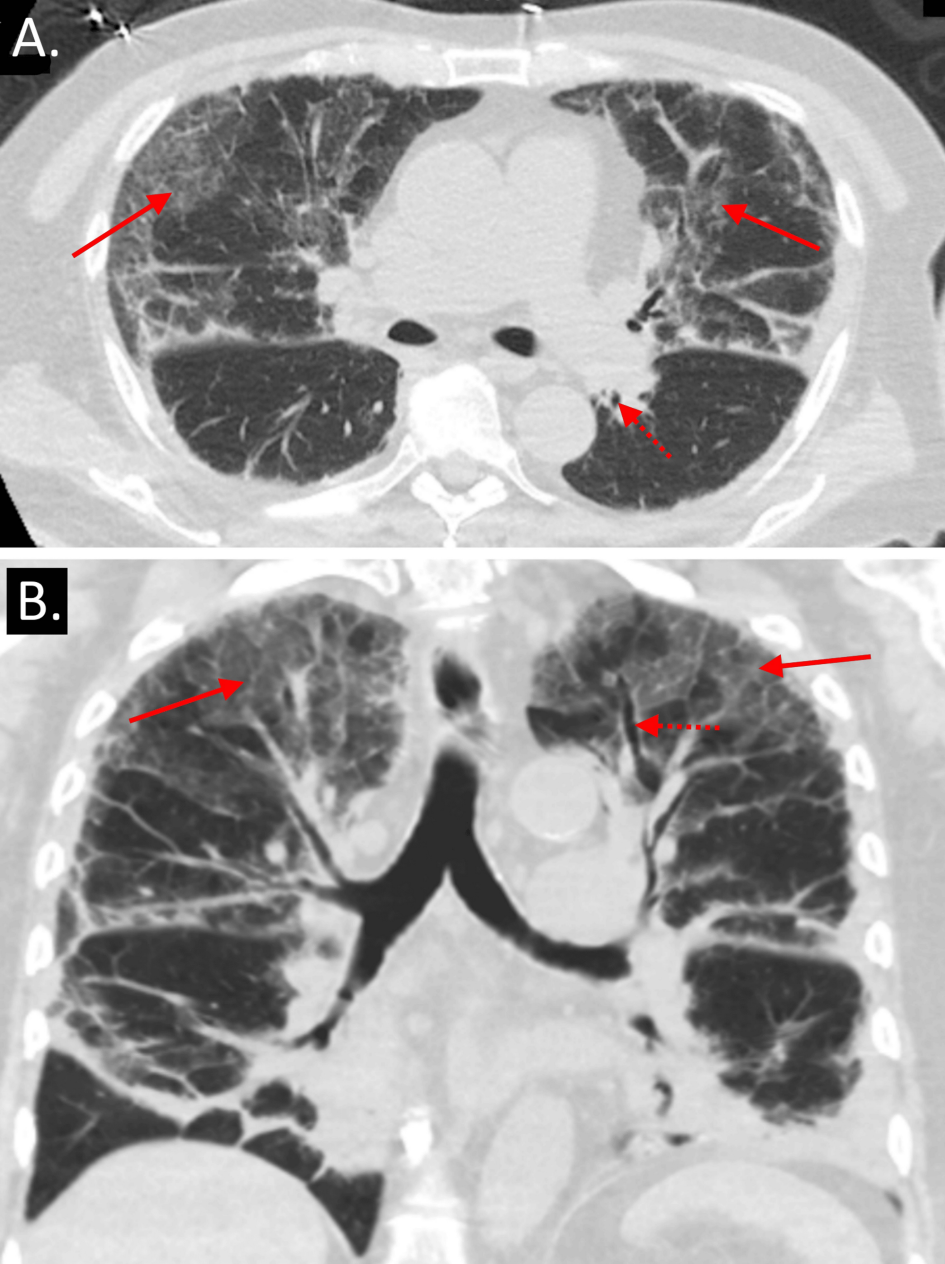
Transverse (A) and coronal (B) views of a non-contrast CT scan of the patient’s chest showing bilateral ground glass opacities (solid arrows) and bronchiectasis (dotted arrows)
